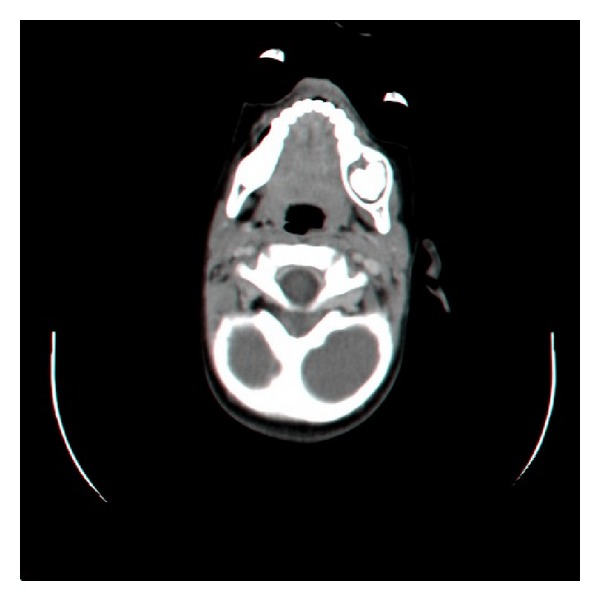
Axial CT of the mandible demonstrates a radiolucent-radiopaque lesion with well-defined borders affecting the left body of the mandible.
